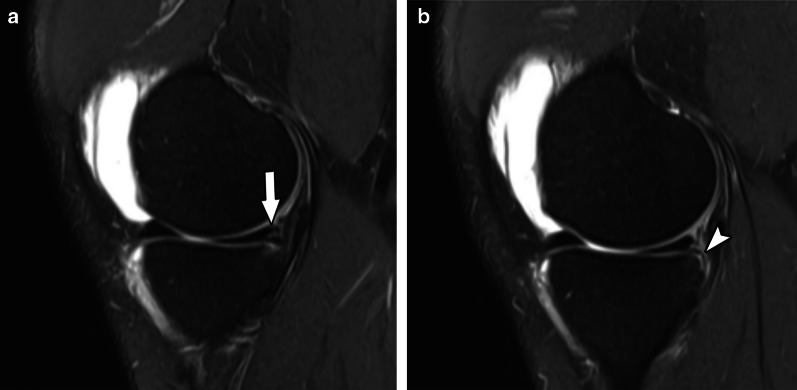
Type 3A meniscal ramp lesion MRI Case. Sagittal T2-weighted fat-suppressed images show peripheral vertical partial tear (arrow in a) extending to the tibial surface of the posterior horn of medial meniscus, with intact meniscotibial ligament (arrowhead in b)
